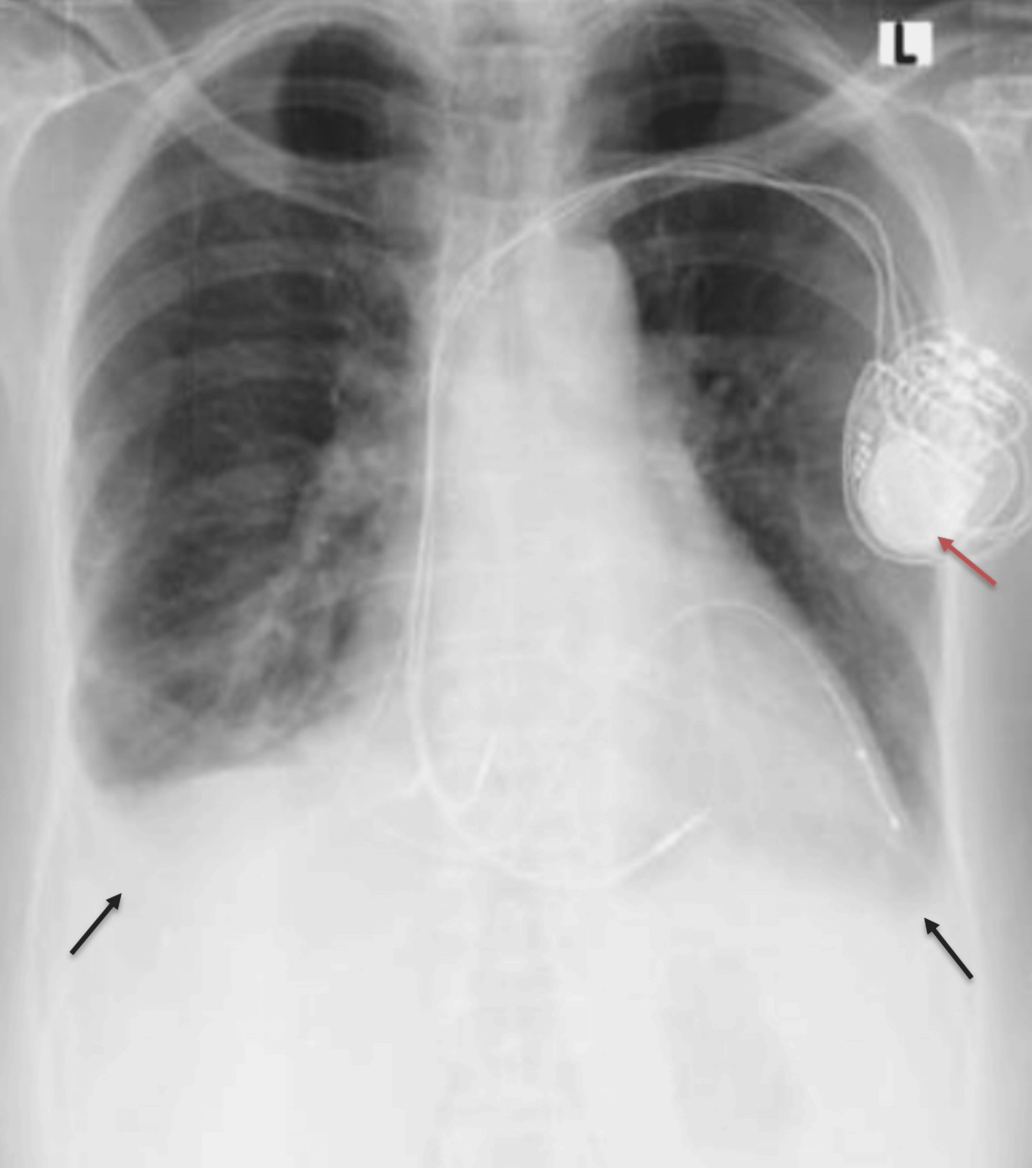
Repeat frontal chest radiograph post cardiac resynchronisation therapy pacemaker (CRT-P) insertion Demonstrates reduction in bilateral pleural effusions (black arrows) and newly inserted CRT-P (red arrow)
